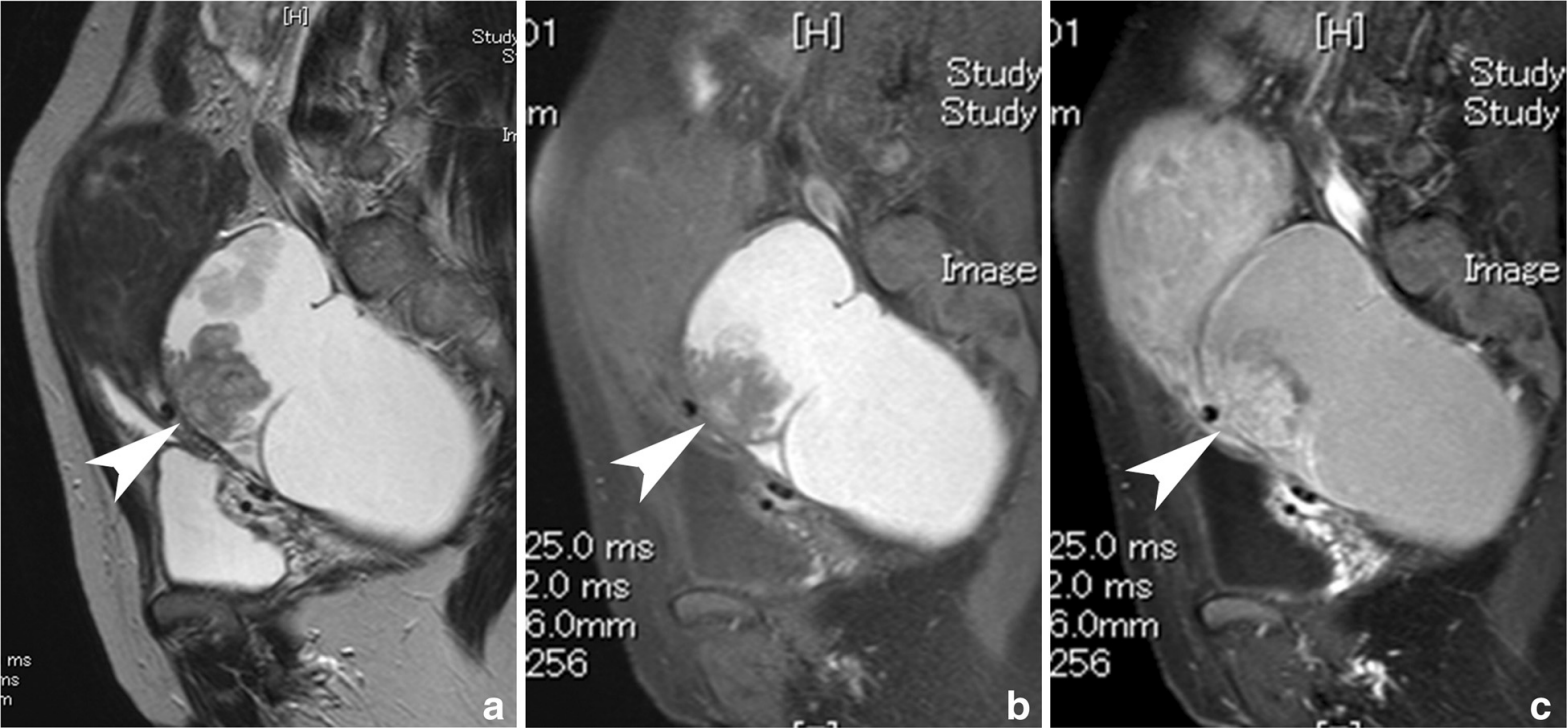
A case with endometrioid carcinoma. A multilocular cystic mass with mural nodules is seen in the cul-de-sac adhering to the uterus on sagittal T2-weighted image (a). The content of the cyst also shows high signal intensity on sagittal fat-saturated T1-weighted image  (b), suggesting co-existing endometriosis. The mural nodules are also well enhanced (arrow heads, c: contrast-enhanced and fat-saturated T1-weighted image). As these imaging characteristics share those of serous and clear cell carcinoma, the specific diagnosis with imaging findings seems the most difficult among 4 subtypes
